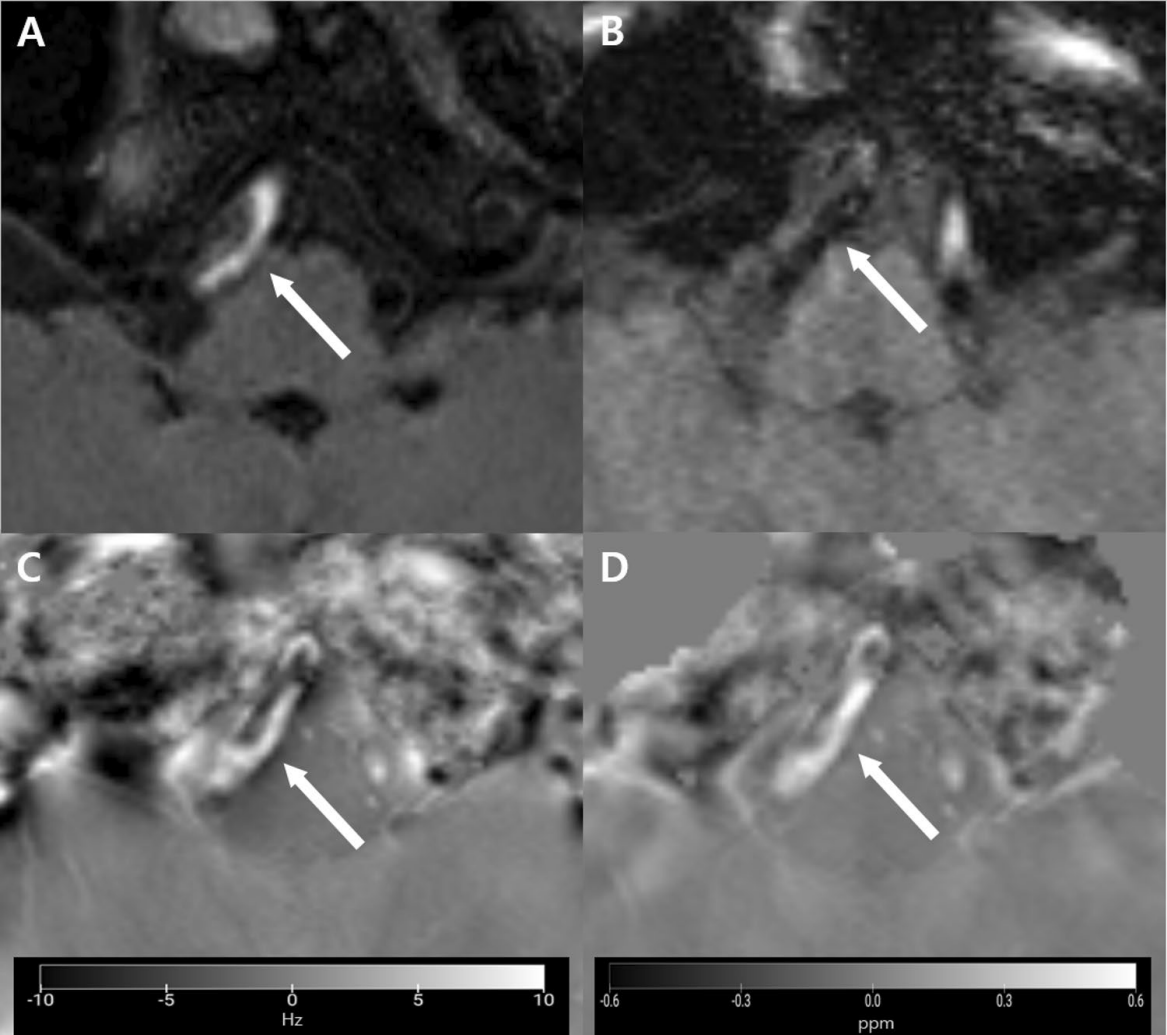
A dissecting intramural hematoma in a 50-year-old male patient. (A) An axial precontrast T1-weighted image of vessel wall magnetic resonance imaging (VW-MRI) shows eccentric T1 hyperintensity (arrows) in the wall of the right intracranial vertebral artery, suggesting dissecting intramural hematoma. (B) A magnitude image shows dark signal intensity of the lesion (arrows). (C) A phase image shows a high signal intensity lesion of the lesion (arrows). (D) The lesion has high susceptibility values (mean, 0.328 ppm; median, 0.319 ppm) on quantitative susceptibility mapping (QSM) (arrows), suggesting the paramagnetic nature of the hematoma. The figures were generated using the MRIcroGL version 1.2.20220720 (https://www.nitrc.org/projects/mricrogl/).
